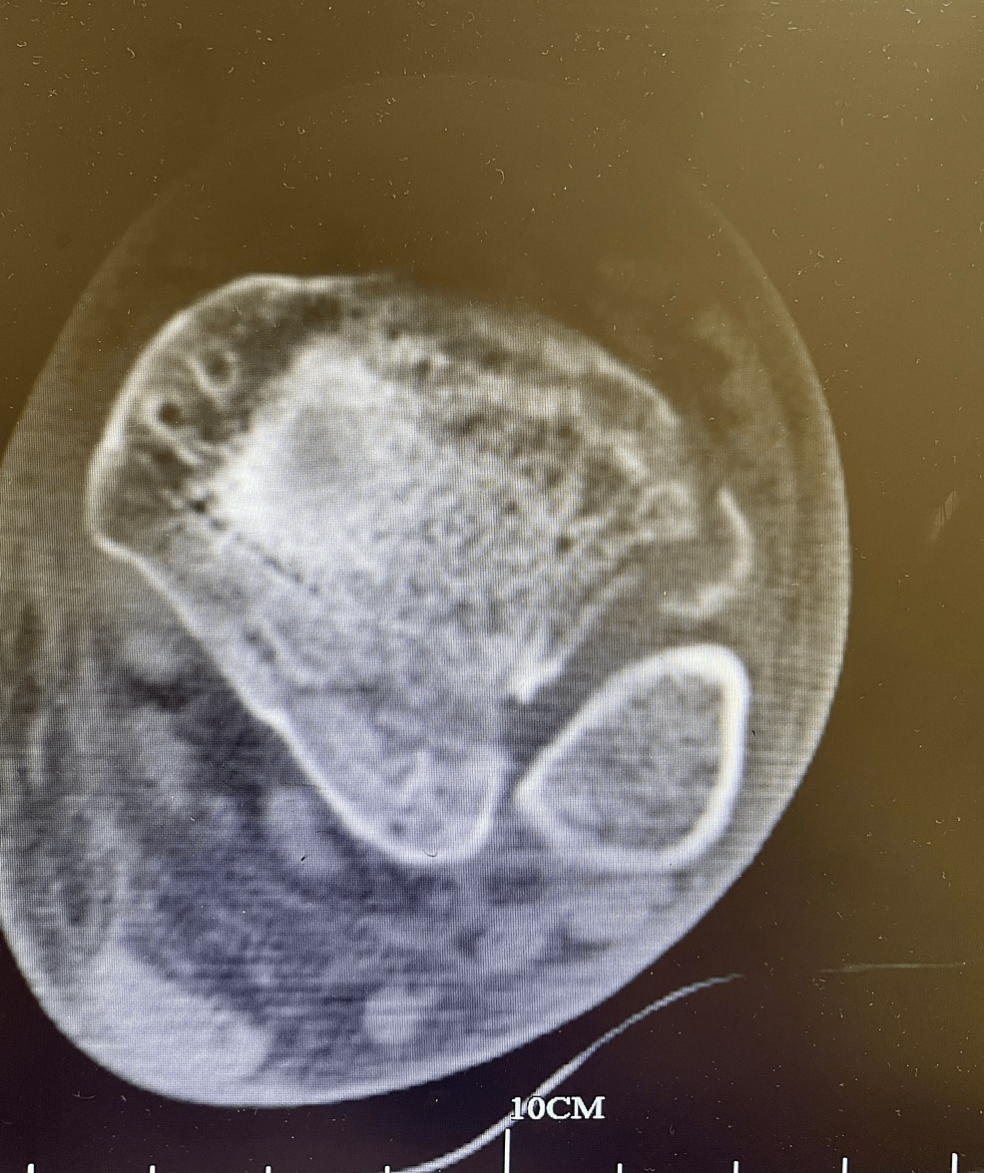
Axial CT scan of the ankle shows the posterior malleolar fracture and anterior malleolar fracture.
